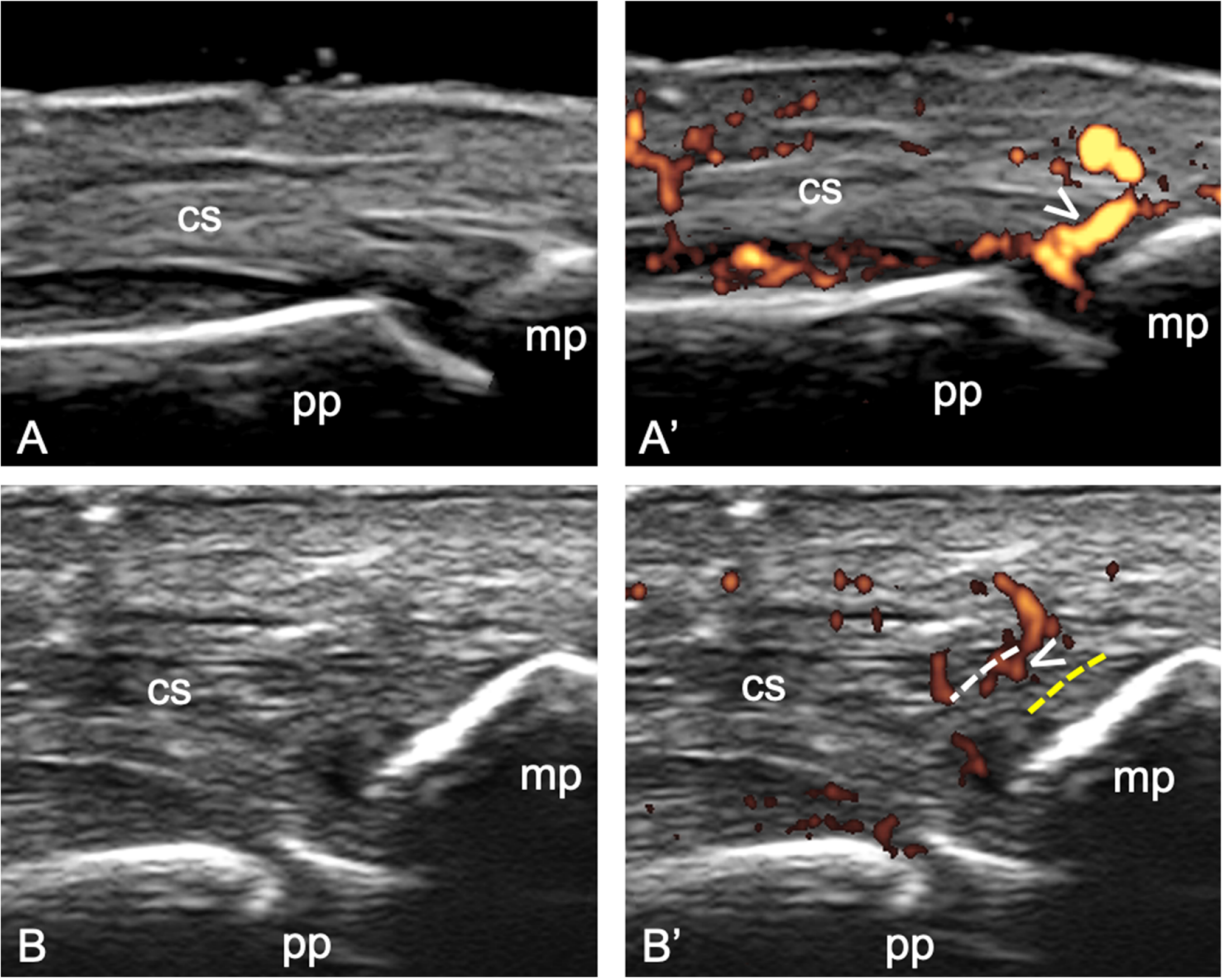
Longitudinal dorsal scans obtained with a 22-MHz probe at the proximal interphalangeal joint level in two psoriatic arthritis patients, without (A and B) and with (A’ and B’) power Doppler mode, respectively. Distinct patterns of vascularization at the central slip (cs) of the finger extensor tendon insertion into the middle phalanx (mp) are shown. In A’, power Doppler signal (arrowhead) is close to the enthesis, while in B’, although being within 2 mm from the bony cortex (dashed line), the pre-insertional region is spared. We hypothesize that the optimal cut-off might be the half of the tendon thickness (yellow dashed line) in order to differentiate these two vascular patterns at small entheses level. pp = proximal phalanx
